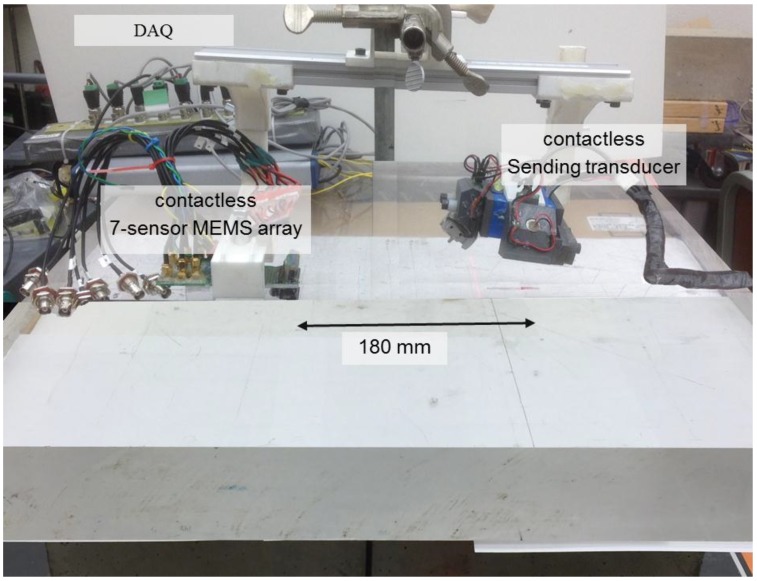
Ultrasonic surface wave testing configuration on the Poly-methyl methacrylate (PMMA) sample; 7-sensor MEMS array used to receive waves.
